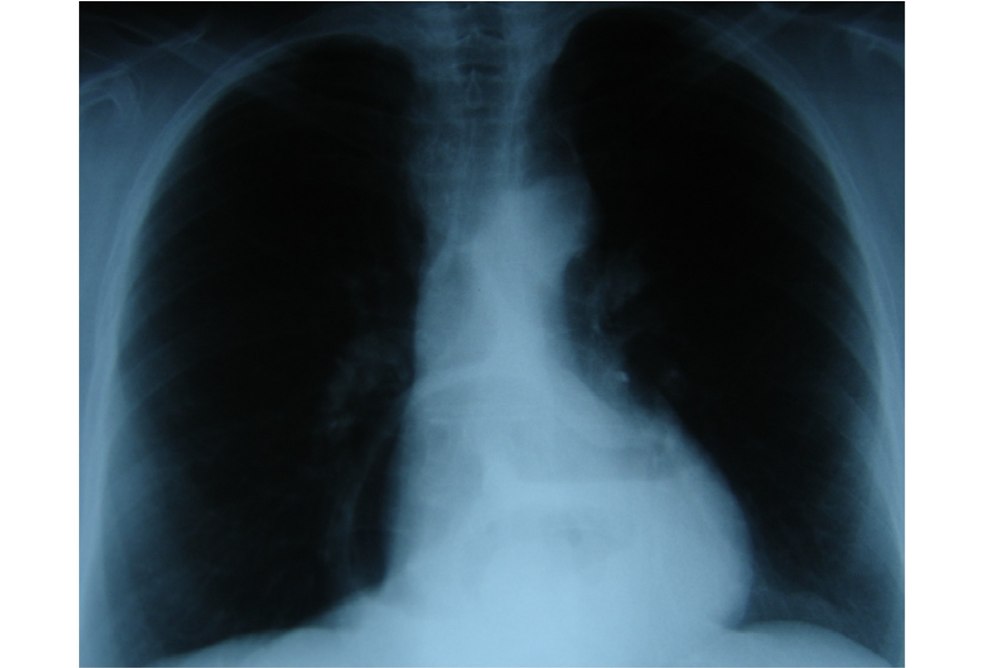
Chest x-ray showing air bubbles in the left chest.
